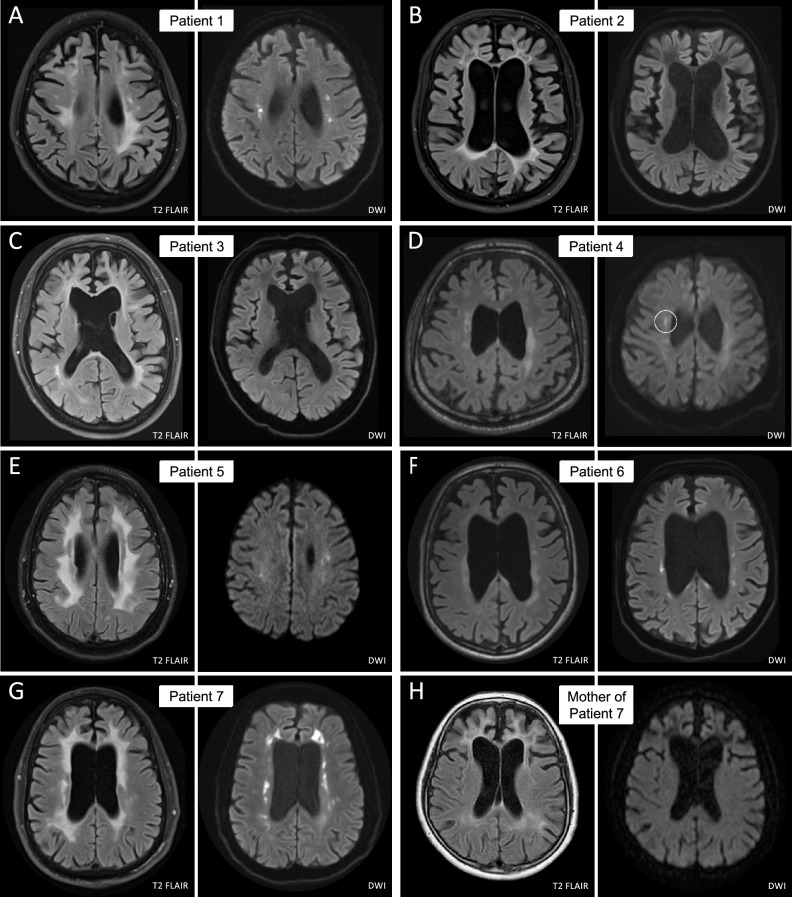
Cerebral magnetic resonance imaging. Magnetic resonance (MR) T2-weighted fluid-attenuated inversion recovery (FLAIR) as well as diffusion-weighted images (DWI) of every patient are depicted. The hallmark features of ALSP in MR imaging are patchy to confluent T2-hyperintensities mainly in the frontoparietal white matter while sparing the temporal lobe, supratentorial global brain atrophy accentuated in the frontal region as well as dispersed foci of restricted diffusion in the periventricular white matter on DWI sequences. All patients had white matter lesions and marked brain atrophy. In five of the patients, diffusion restriction was detected (Patients 1, 4 (circle), 5, 6, 7). All sites of diffusion restriction are hypointense in the apparent diffusion coefficient (ADC) sequence (data not shown)
